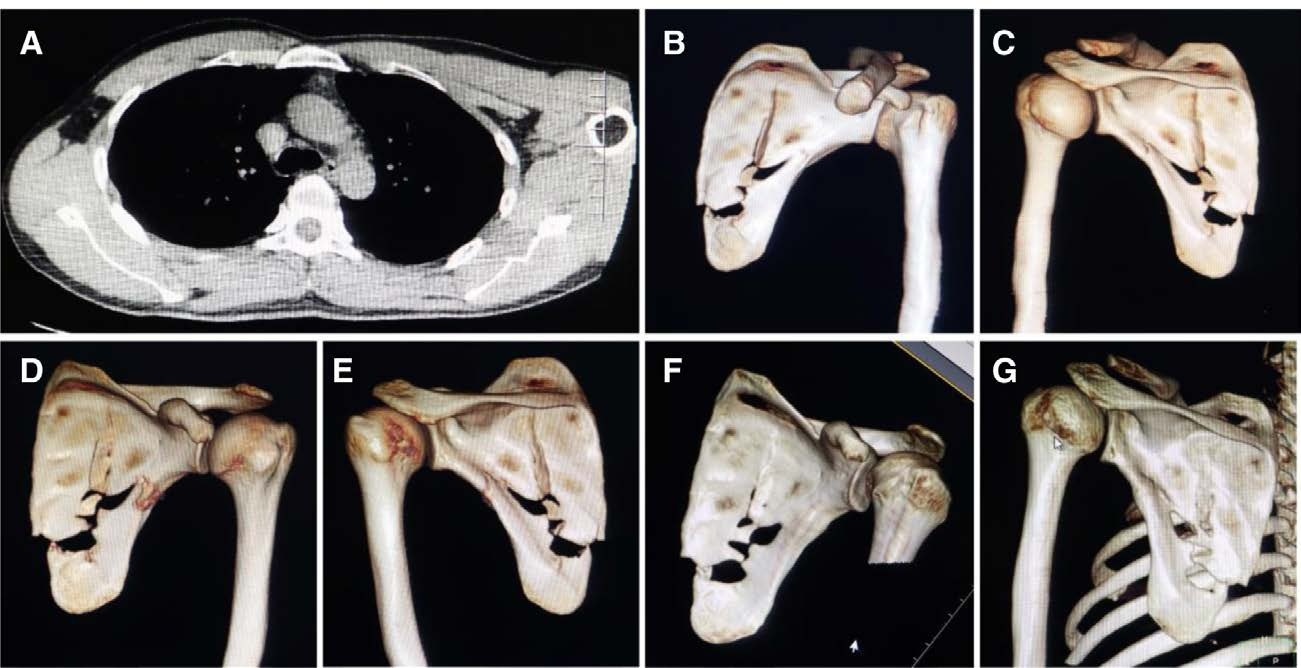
Partial examination results of case 2. (A) Chest CT scans suggested a fracture of the left scapula without muscle tear or hematoma. (B, C) CT 3-dimensional reconstruction pointed to multiple fractures of the left scapular body with displacement of the broken ends, the joints were not involved. (D, E) Calluses were more than before on reexamination 18 days from injury. (F, G) 3D reconstruction images at review 3 months after the accident. CT = computed tomography.
